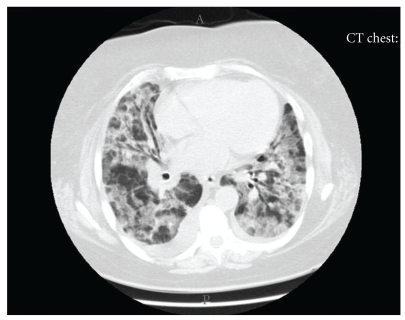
High resolution CT of the chest showed diffuse ground glass opacities mainly involving the upper lobes.
